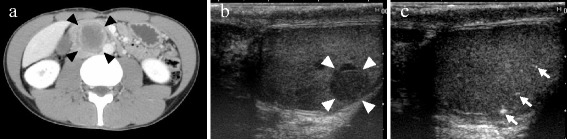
a Computed tomography shows a retroperitoneal tumor located between the aorta and the inferior vena cava. The size of the tumor (*arrowheads*) was 52×36×31 mm, and the testicular tumor was not detected in computed tomography. b Ultrasound examination revealed a tiny low echoic tumor (*arrowheads*) and a low echoic mosaic pattern around the tumor. c Microcalcifications of various sizes around the tumor were detected in ultrasound (*arrows*)
